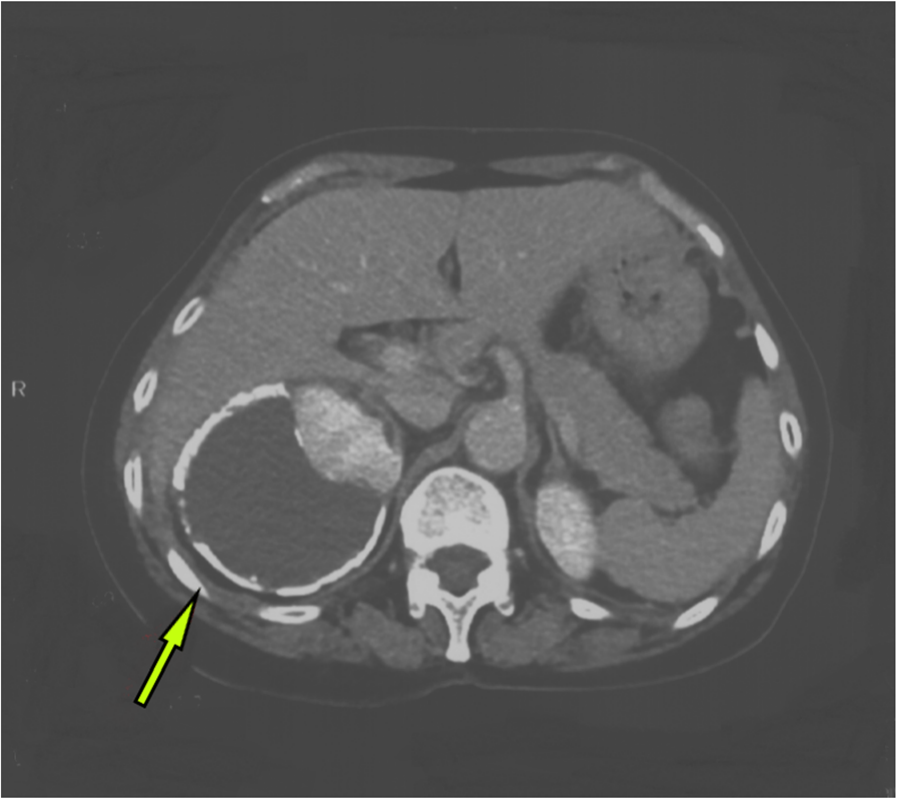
Enhanced CT scan showing a circular low-density shadow under the annular calcification wall
